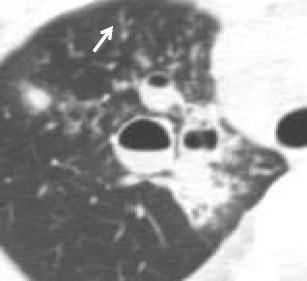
Twenty-one-year-old man with axial CT image demonstrating saccular bronchiectasis with tree-in-bud opacity (*arrow*), debris, and mucous plugging
